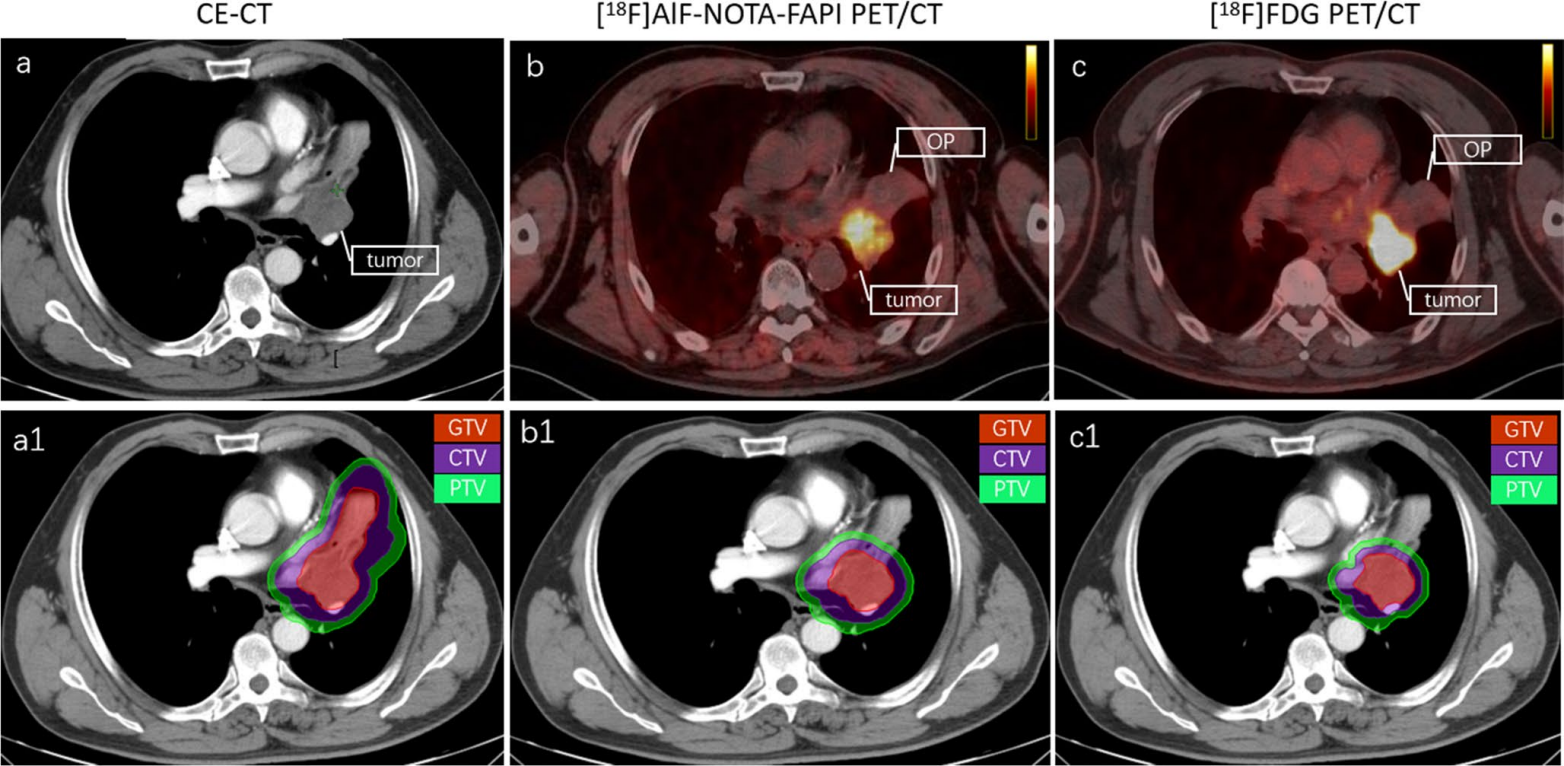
A 63-year-old man was diagnosed with central lung cancer complicated with obstructive pneumonia (OP). Both [^18^F]AlF-NOTA-FAPI and [^18^F]FDG PET/CT imaging showed clearly boundary of tumors and OP, and then more accurate and small-volume GTVp, CTVp and PTVp can be delineated. CE-CT images are difficult to distinguish the boundary between the tumor and OP, and delineated GTVp, CTVp and PTVp are larger than the true tumor volume
